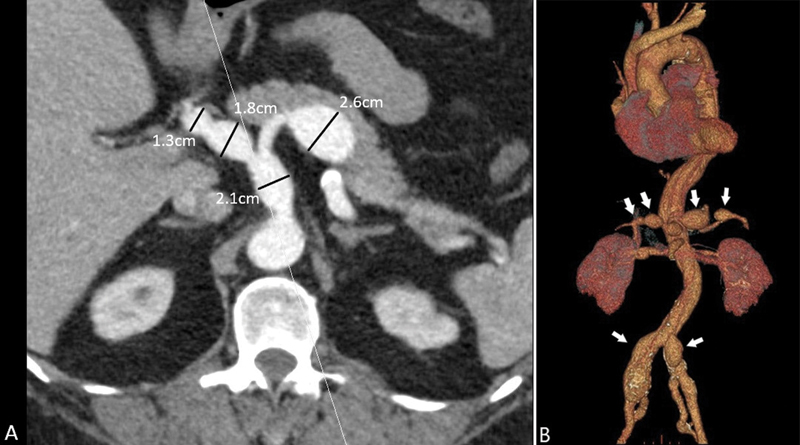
Computed tomography angiography images showing multiple artery aneurysms: (
